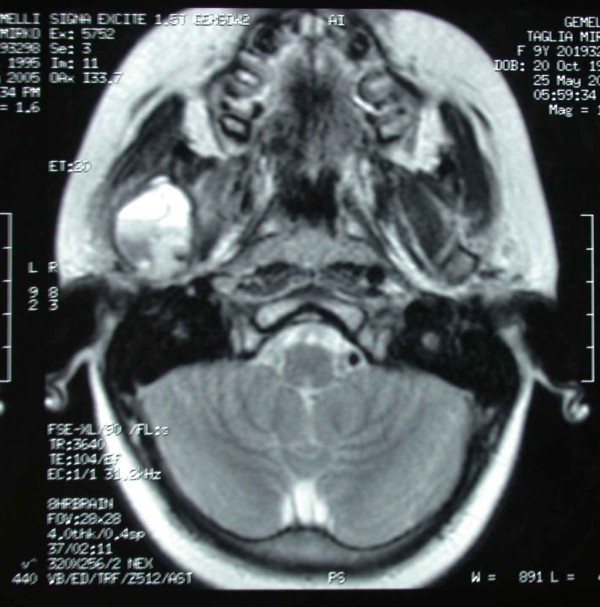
Magnetic resonant imaging (MRI) showing a high-signal intensity within the lesion itself, with a low-signal intensity at the periphery.
